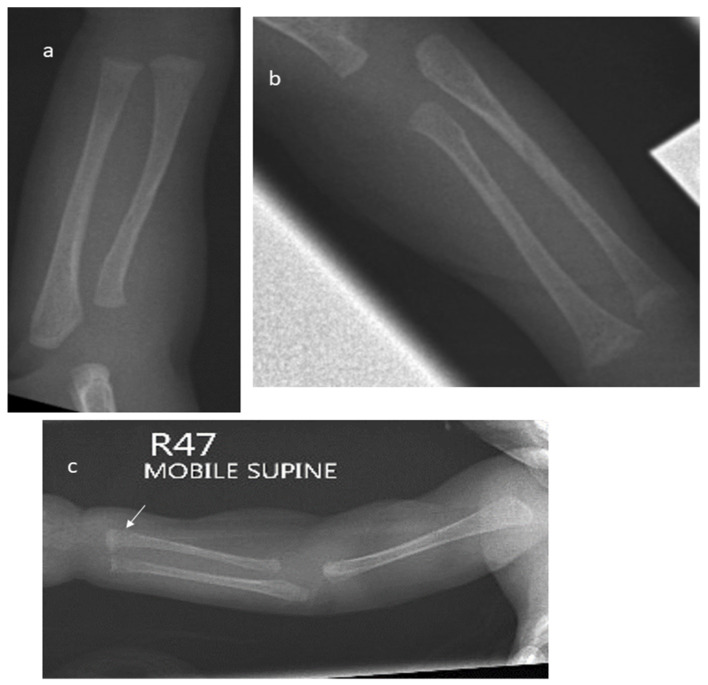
(a) X-ray of the right forearm in a 6-week-old infant born at 26 weeks showing changes of Grade 1 MBDP. (b) Wrist X-ray of an 8-week-old female infant born at 25 weeks showing cupping and fraying of metaphyses, Grade 2 MBDP. (c) X-ray of the right forearm in an 8-week-old infant born at 24 weeks showing a fracture at the distal end of the radius (marked by arrow).
